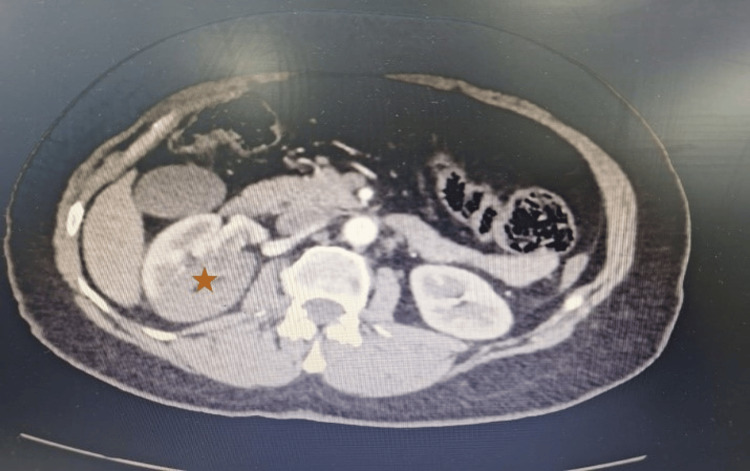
CT of the abdomen and pelvis with intravenous contrast demonstrating a wedge-shaped area of hypoenhancement with abrupt occlusion of the posterior segmental artery and a positive cortical rim sign, consistent with acute renal infarction (indicated by an asterisk).
